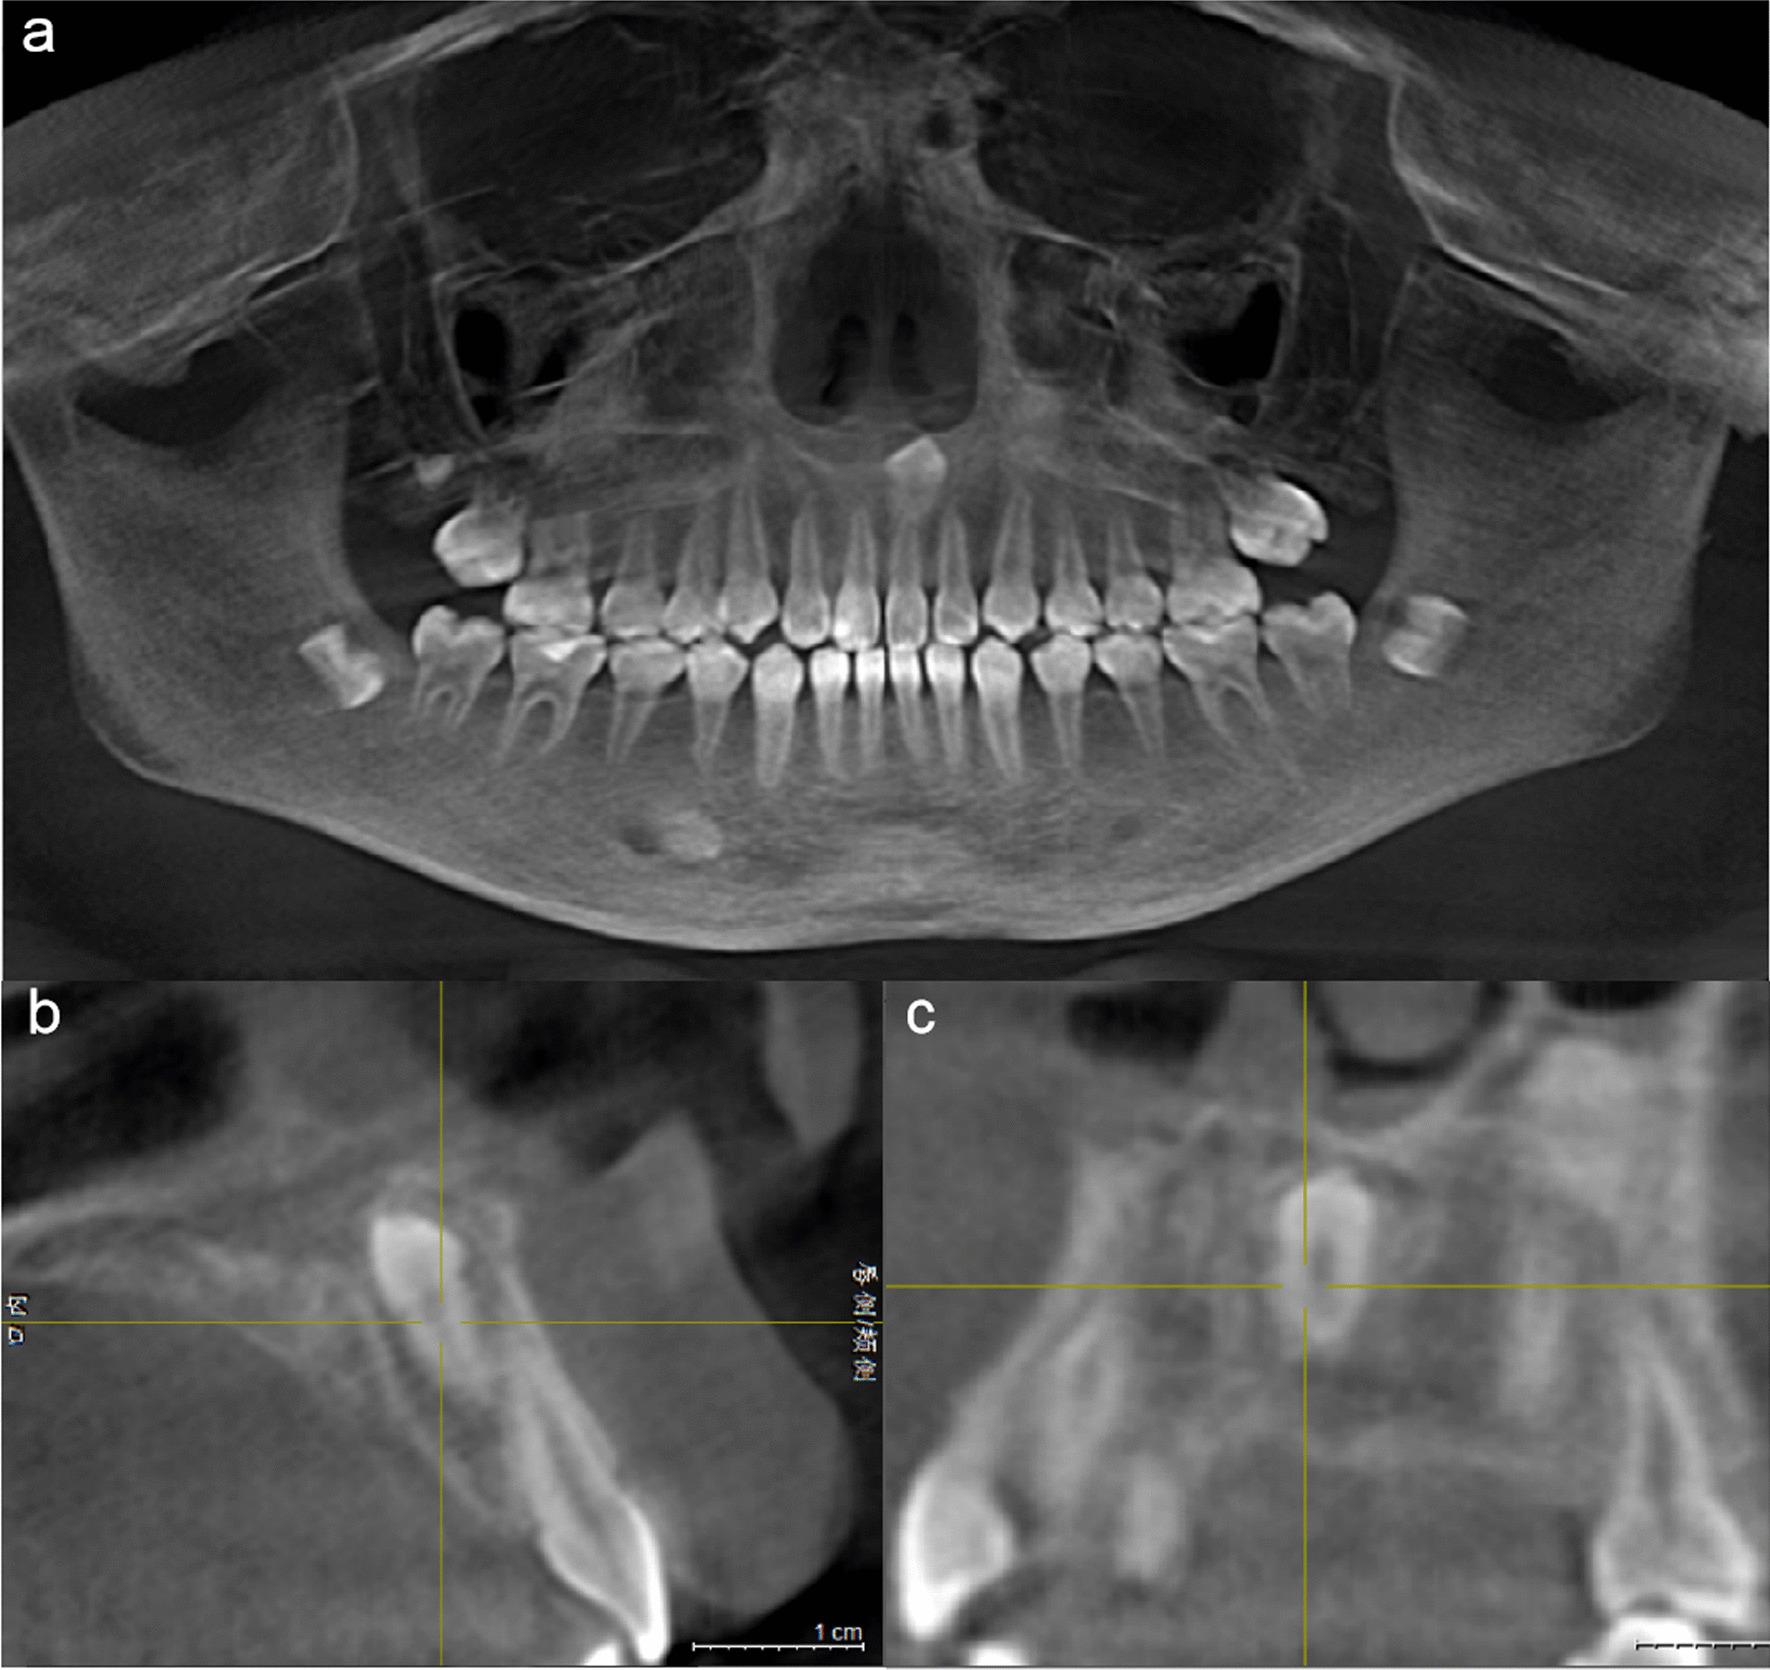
Pre-operative CBCT data of the patient. a Preoperative CBCT picture shows that: an inverted embedded supernumerary teeth can be seen above the 21 apex, close to the nasal cavity; b Crown position; c Horizontal position
